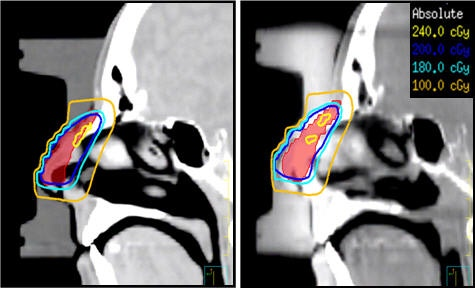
A sagittal dose distributions for the reference CT data (left) and of a CBCT dataset (right). The PTV is shown as a wash contour. The isodose lines shown correspond to the 120%, 100%, 90% (Rx line), and 50% isodose lines.
